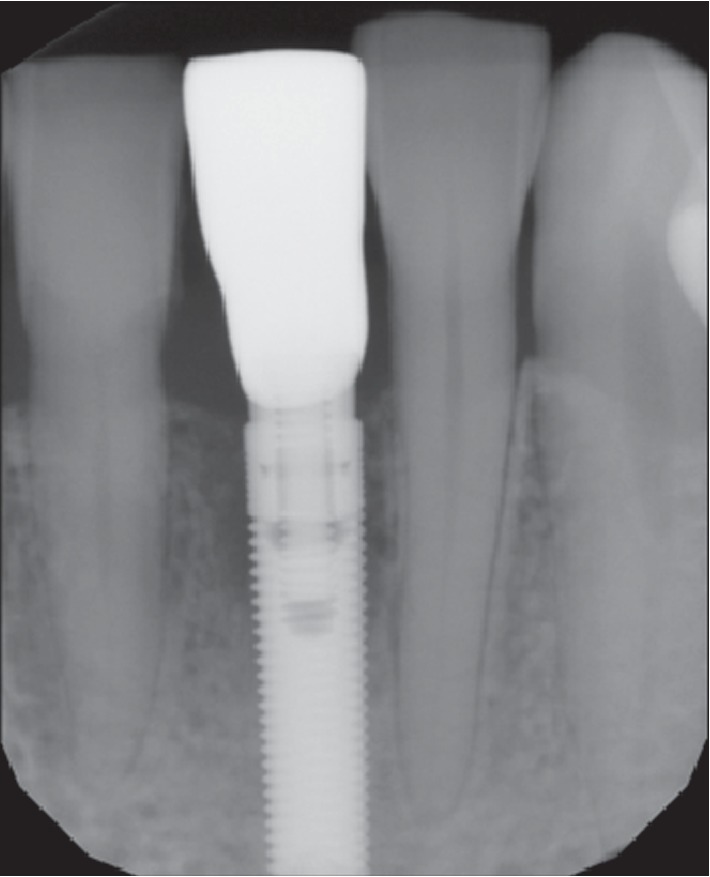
Case 2: radiographic view of final restoration.
